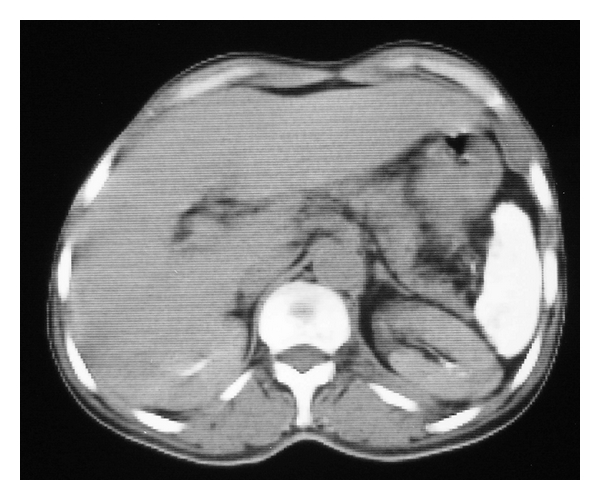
Abdominal CT scan showing a small atrophied and calcified spleen (autosplenectomy).
